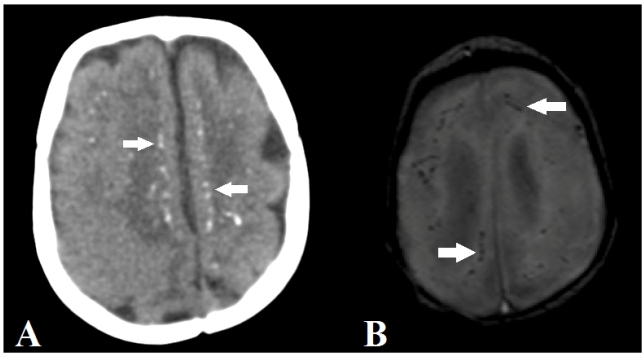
Axial computed tomography (A) and magnetic susceptibility
